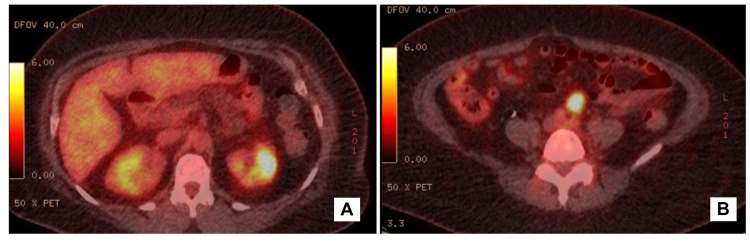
Lesion as observed on the skull base to mid-thigh FDG-PET scanning before the surgical intervention (A) Hypermetabolic lesion in the upper pole of the left kidney. (B) Hypermetabolic inferior mesenteric lymph node FDG-PET, fluorodeoxyglucose-positron emission tomography
